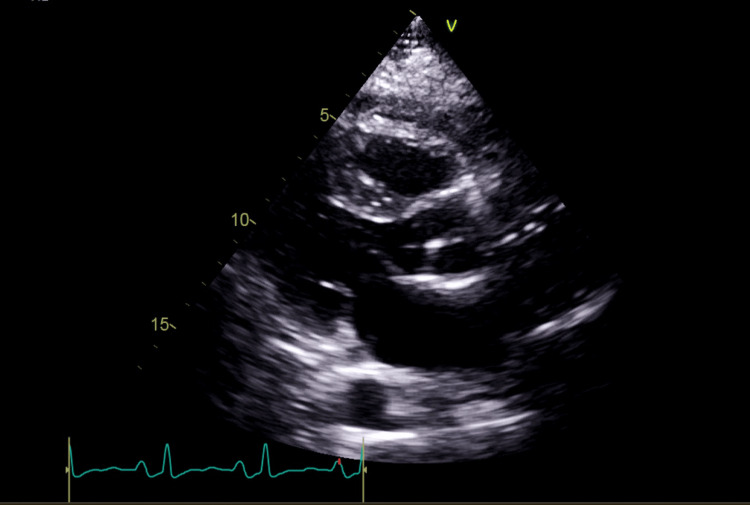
Echocardiography after pericardiectomy showing mild to moderate pericardial effusion without compressing the heart chambers
